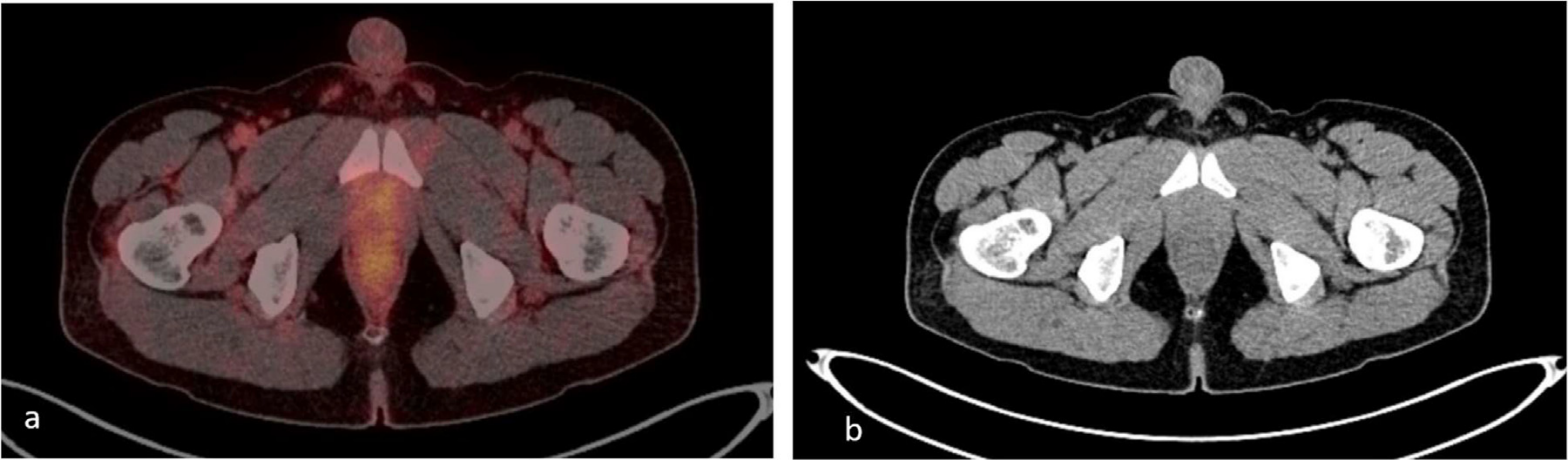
(A) Axial PSMA PET/CT imaging of the prostate and (B) axial CT imaging of the prostate.
